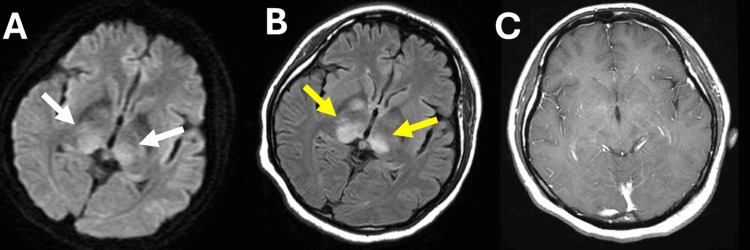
Initial magnetic resonance imaging findings. A) A diffusion-weighted imaging sequence revealed high-signal intensity areas (white arrows) in bilateral basal ganglia. B) A fluid-attenuated inversion recovery image revealed high-signal intensity areas (yellow arrows) in bilateral basal ganglia. C) No contrast enhancement was observed on a gadolinium T1-weighted image.
